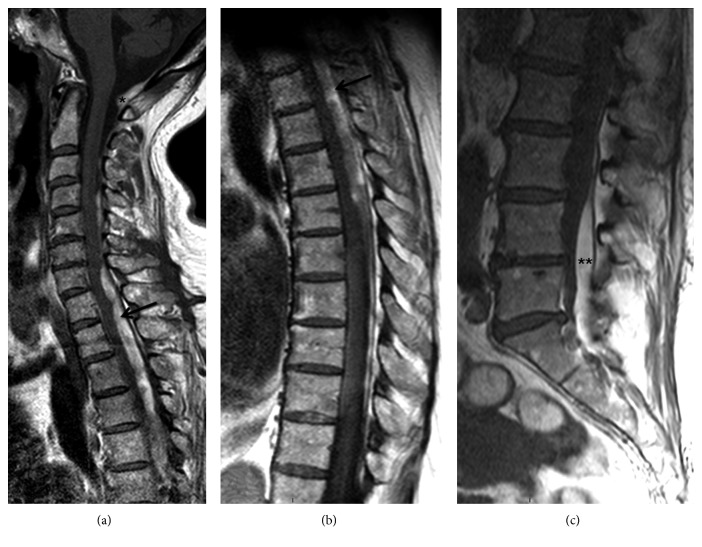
MRI scans: T1 sagittal view of the cervical (a), dorsal (b), and sacral (c) column showing a large subacute intraspinal extramedular hematoma from the craniocervical (^∗^) region to the sacral (^∗∗^) region, with spinal cord compression at the dorsal level (→). It was not possible to study the whole column in one sequence due to clinically silent dorsolumbar scoliosis.
